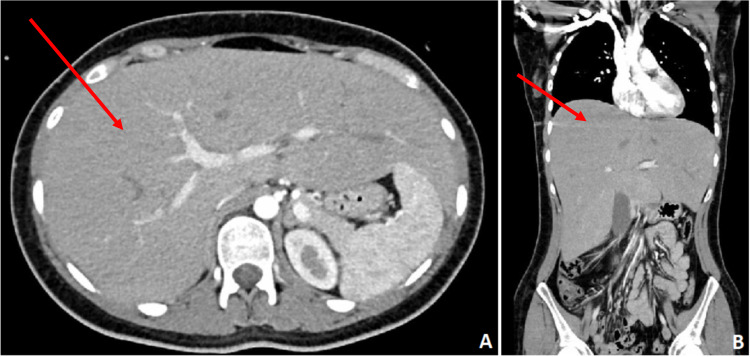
Cross (A) and coronal (B) sections of abdominopelvic computed tomography angiography revealing hepatomegaly
